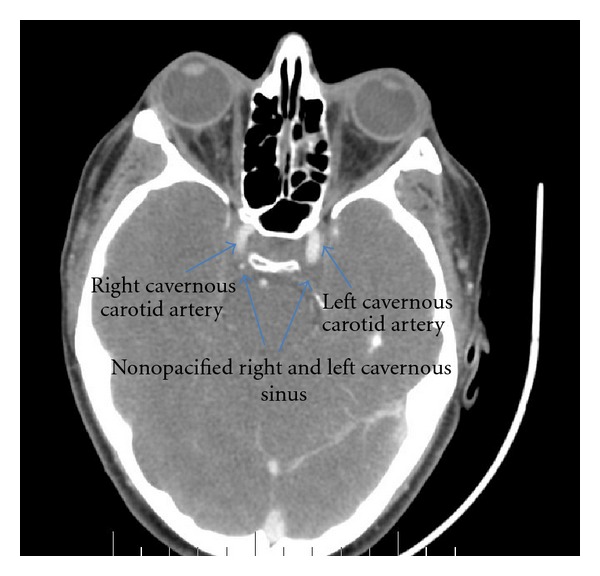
CT scan of the head and neck with contrast demonstrated a thrombus bilaterally into the cavernous sinuses.
